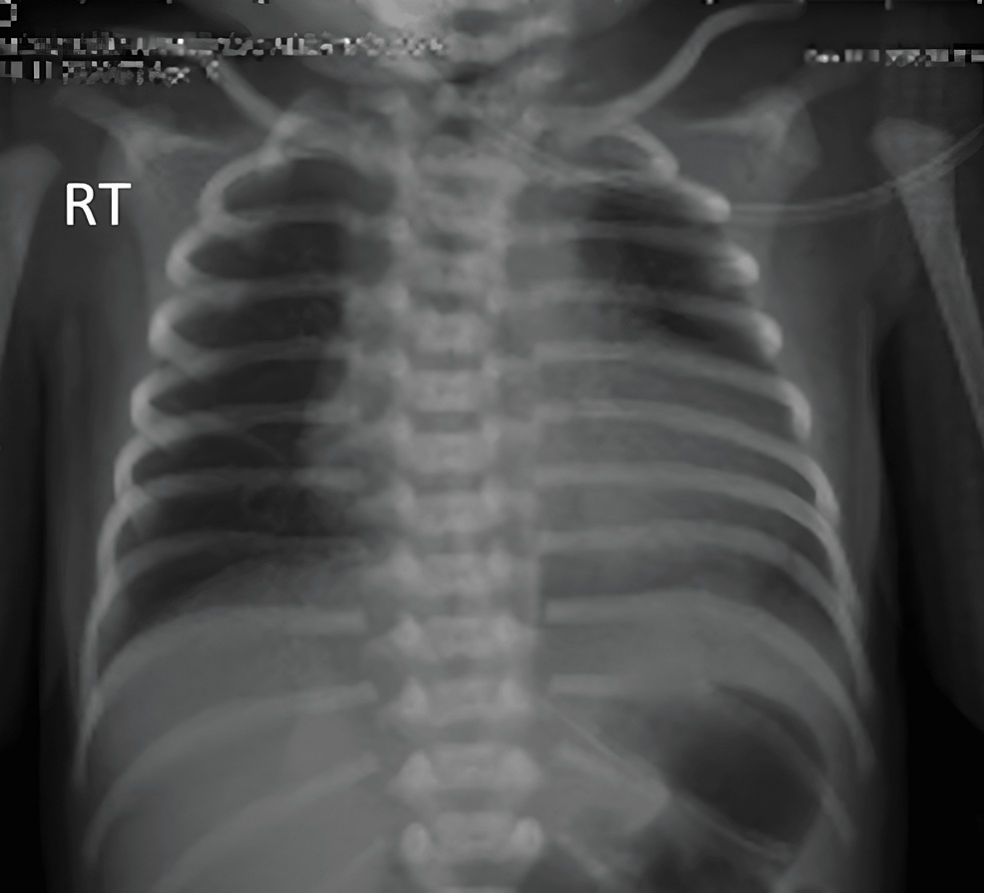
Chest X-ray during the first few hours of life showed mild increased interstitial markings in both lungs
